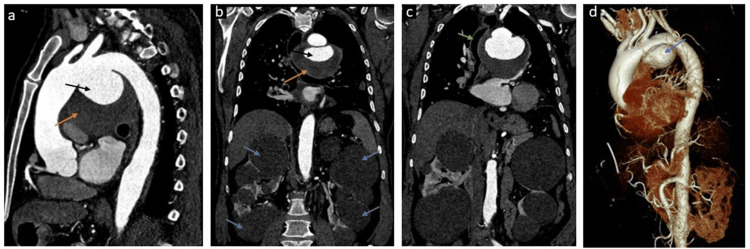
Computed tomography angiograms demonstrating a giant aortic arch aneurysm in polycystic kidney disease patient (A) Sagittal image demonstrating a large aneurysm from the inferior surface of the arch of aorta (black arrow) and large intramural thrombus (orange arrow). (B) Coronal image demonstrating the same (black and orange arrows) and multiple variable-sized bilateral simple renal cortical cysts (blue arrows). (C) Coronal image showing rightward tracheal shift (green arrow) due to mass effect by the aneurysm. (D) 3D virtual rendering technique (VRT) image demonstrating aortic arch aneurysm (blue arrow).
